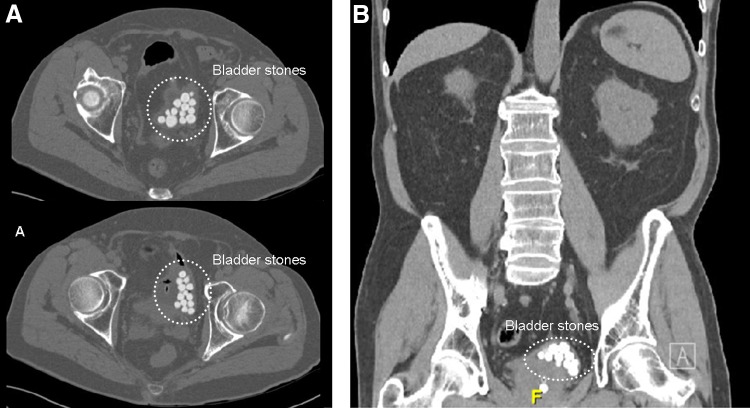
Computed tomography scan showing multiple uric acid bladder stones. (A) Axial view; (B) coronal view.
